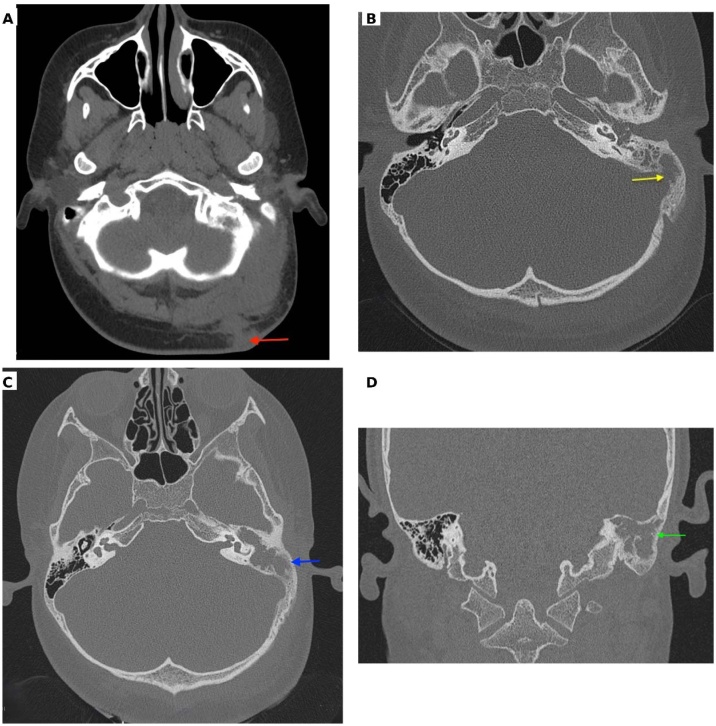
(A) Axial CT scan showing a soft tissue swelling at occipital region (red arrow). (B) Axial CT scan (bone window) showing lesion in the mastoid bone with erosion of the sinus plate (yellow arrow). (C) Axial CT scan showing intact cortical bone (blue arrow). (D) Coronal CT scan showing intact cortical bone (green arrow).
